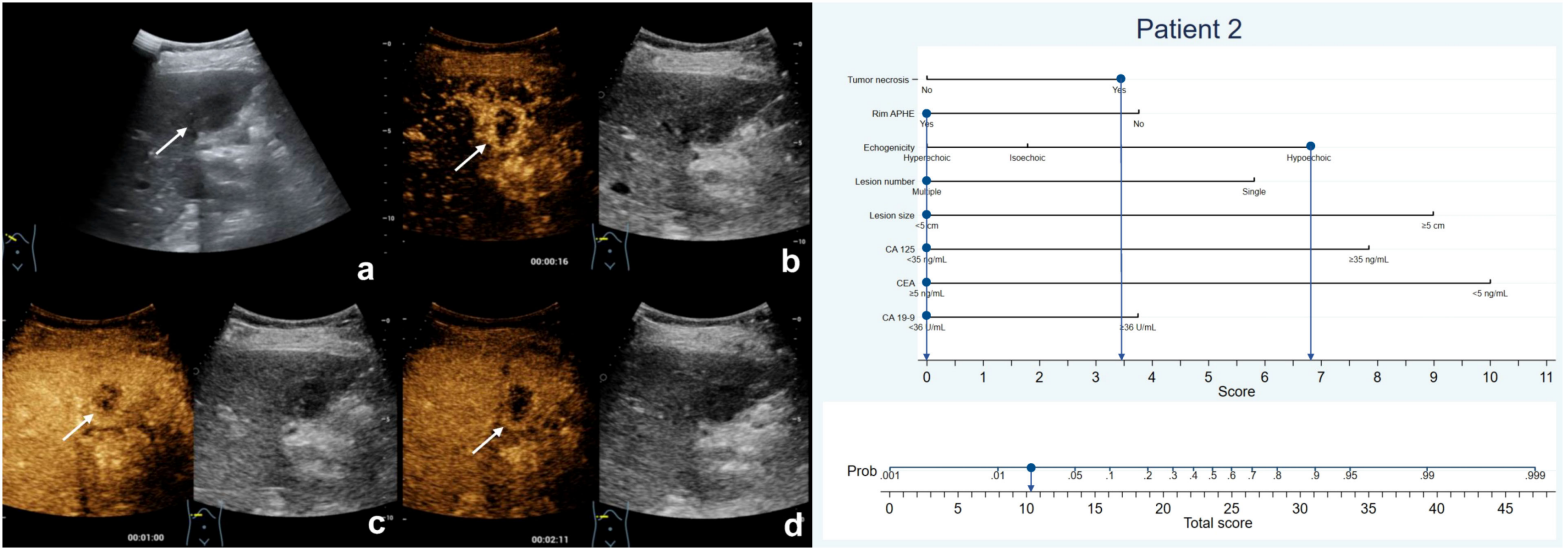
The patient was a 75-year-old female who presented with several hypoechoic lesions in liver, with the largest one measuring 2.5-cm in diameter (A). Rim hyperenhancement of the tumor was observed during the arterial phase (B), and contrast-enhanced ultrasound revealed necrotic areas within the tumor (B–D). The patient exhibited elevated serum CEA levels but normal CA19-9 and CA125 levels. The nomogram score assigned to the patient was 10.2, indicating a less than 10% chance of developing MF-ICC. Pathological analysis confirmed liver metastatic adenocarcinoma originating from colon.
